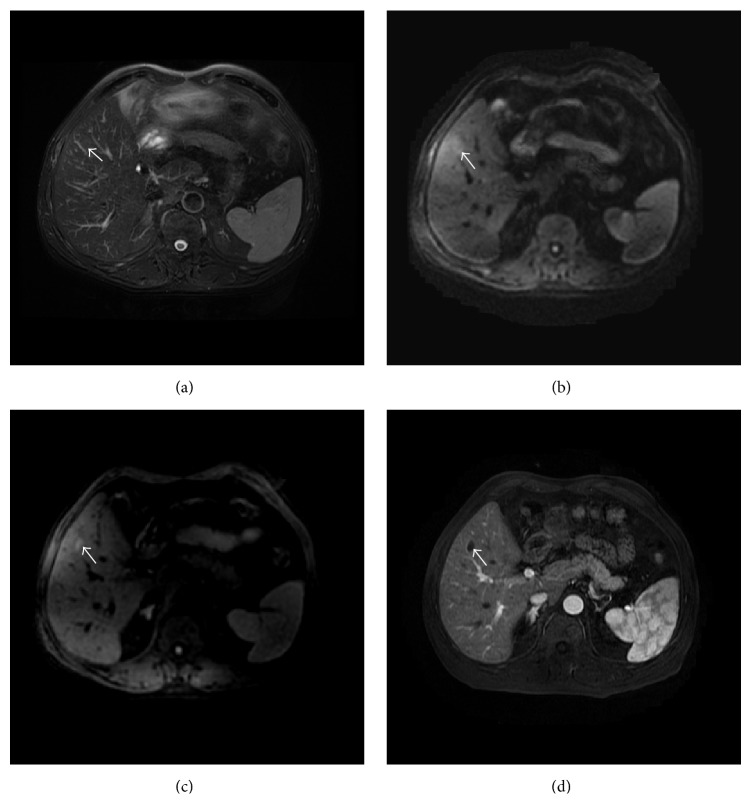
Transverse images in 56-year-old man with solitary necrotic nodule. (a) Fat-suppressed T2-weighted fast spin-echo image shows that lesion is isointense to the liver. (b) DW image with higher *b*-value shows that lesion is difficult to be identified. (c) DW image with low *b*-value shows lesion of hyperintensity could easily be detected. (d) Contrast-enhanced T1-weighted image in arterial phase demonstrates nonenhancement of lesion.
